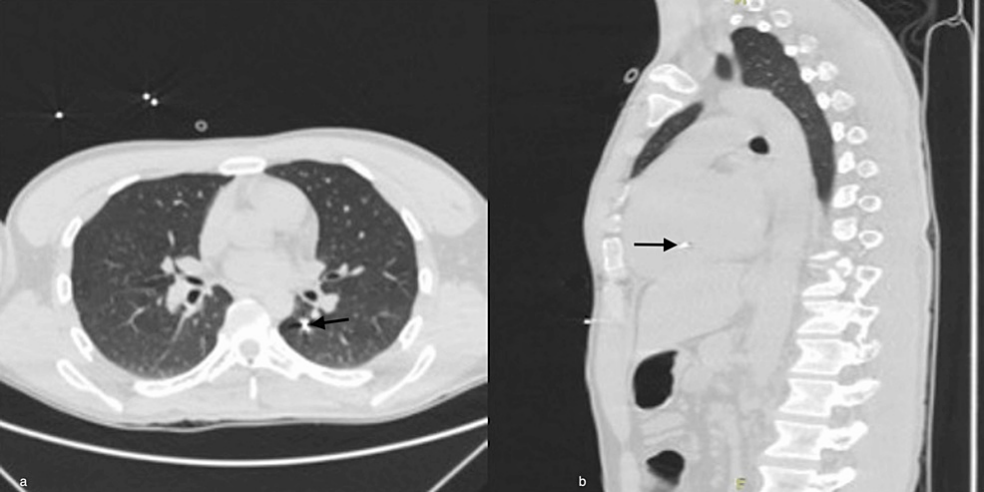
Chest computed tomography at admission. (a) The image on the left reveals a non-thrombotic embolism of metal fragments in the pulmonary artery for the apical segment of the left upper lobe and right intraventricular regions (arrow). (b) On the right, cardiac chambers of normal size are observed with metal fragments inside the right ventricular cavity (arrow).
